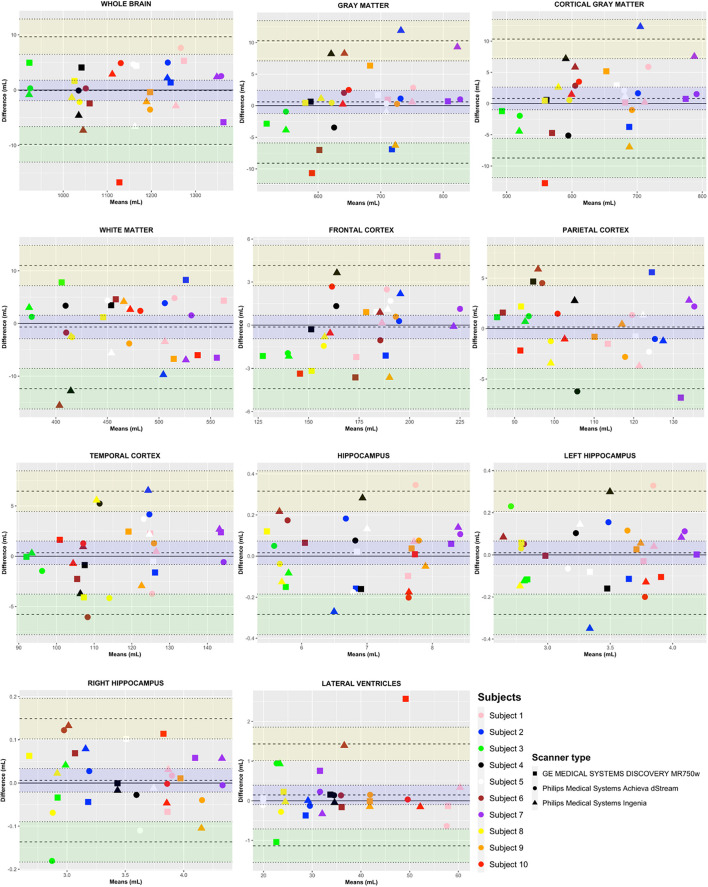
Bland–Altman plots for individual brain structures. Variability results (intra- and inter-scanner, as well as intra- and inter-subject variability) presented in a Bland–Altman plot per brain structure for three different MRI systems; Philips Medical Systems Achieva dStream 1.5T, Philips Medical Systems Ingenia 3T, and GE Discovery MR750w 3T, computed by icobrain dm. The *y*-axis represents the difference in mL [test (scan 1) – retest (scan 2)]. The *x*-axis represents the mean in mL of scan 1 and scan 2 [(scan 1 + scan 2)/2]. The quantitative measurements are presented per subject (color-coded differentiation) and per scanner (symbol-coded differentiation). Here, the limit of agreement (LOA, upper LOA in yellow and lower LOA in green) represents the 95% prediction interval [1.96 SD].
